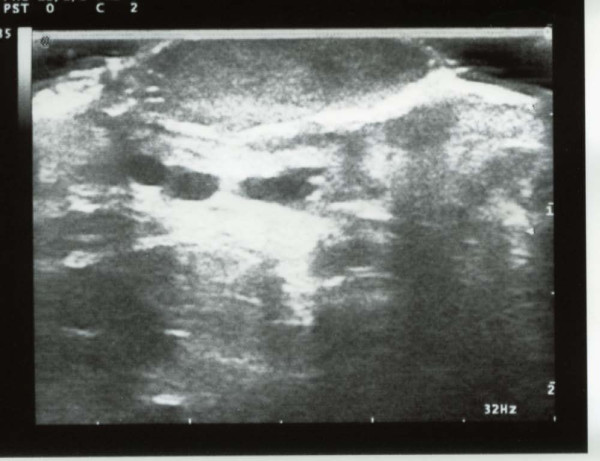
Ultrasound image of a nodule in a patient with Classic Kaposi Sarcoma. The formation is homogeneous, hypoechoic, with clear and well-defined contours. It involves the epidermis and derma and it is associated to ectasia of local-regional vessels in adipose sub-cutaneous tissue.
